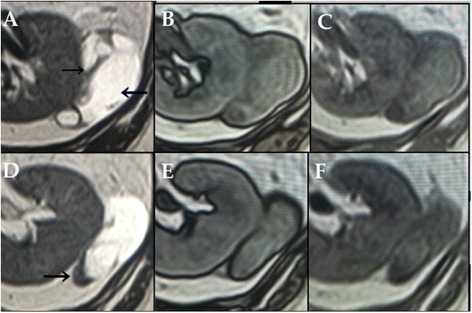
Preoperative magnetic resonance imaging (MRI). On T2-weighted MRI, the cystic mass and septal wall appear as hyperintense and hypointense areas, respectively. Moreover, the continuity between the renal parenchyma and the cystic mass is not clear (a, d – black arrows). T1-weighted MRI does not show a signal change between the in-phase (c, f) and out-of-phase (b, e) images, indicating that the cystic mass has no adipose tissue
